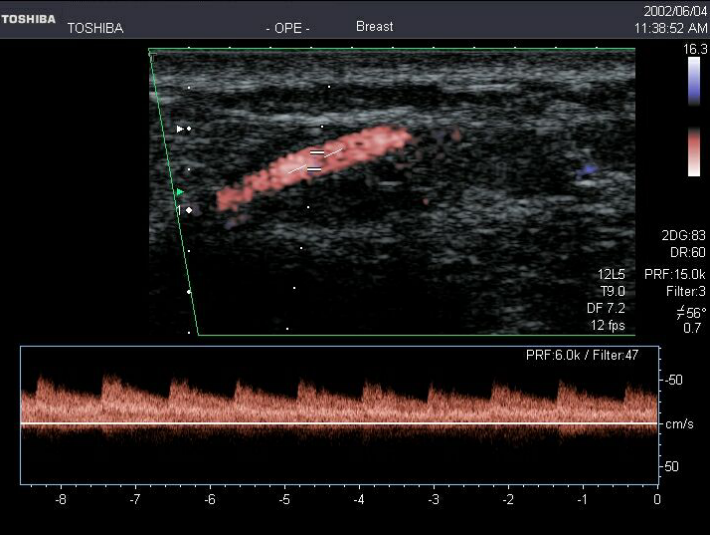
The origin of the anterior mammary branch of the Internal Mammary Artery imaged with Colour Flow Doppler Imaging.
